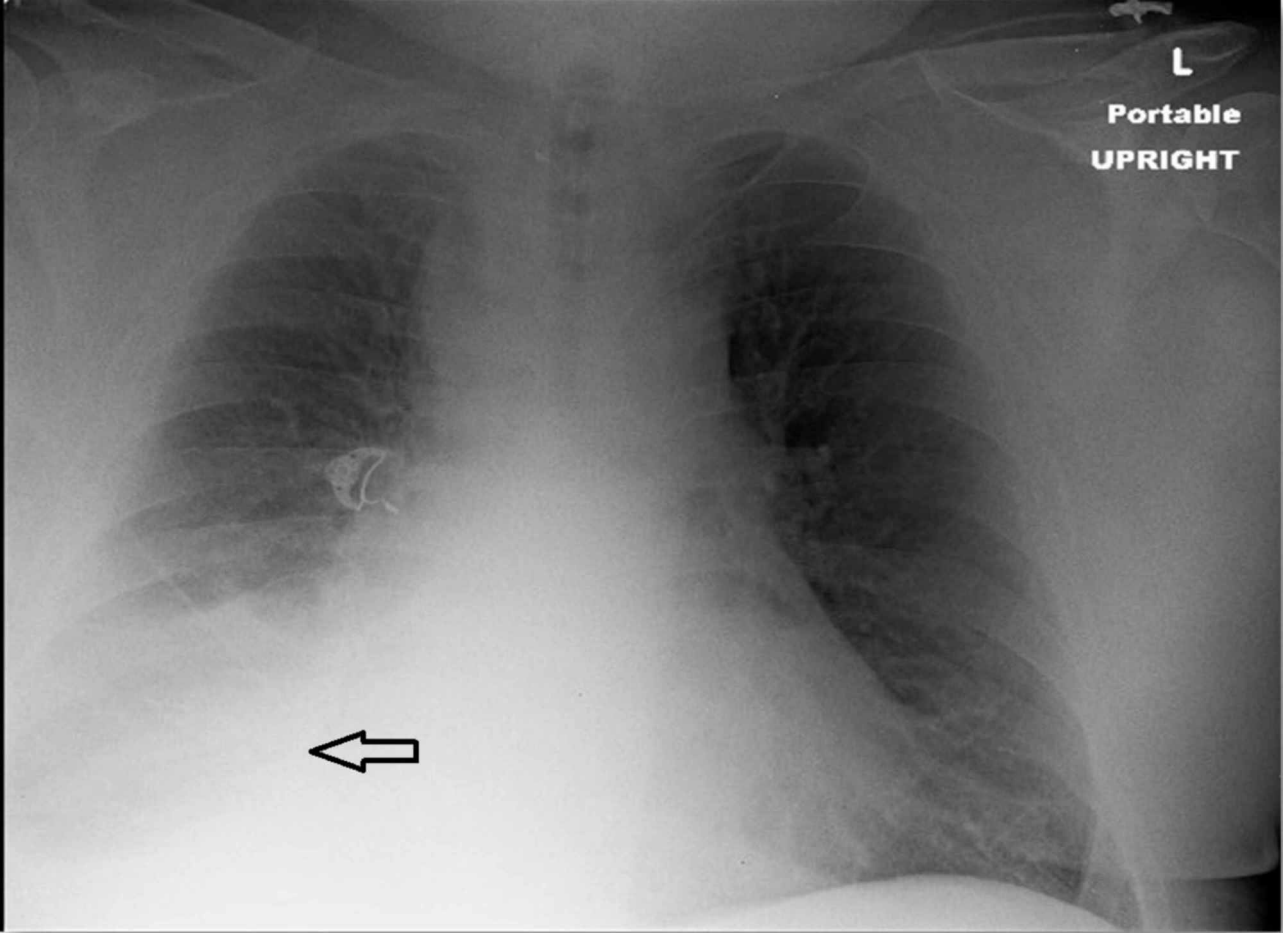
Initial chest X-ray showing right-sided pleural effusion
